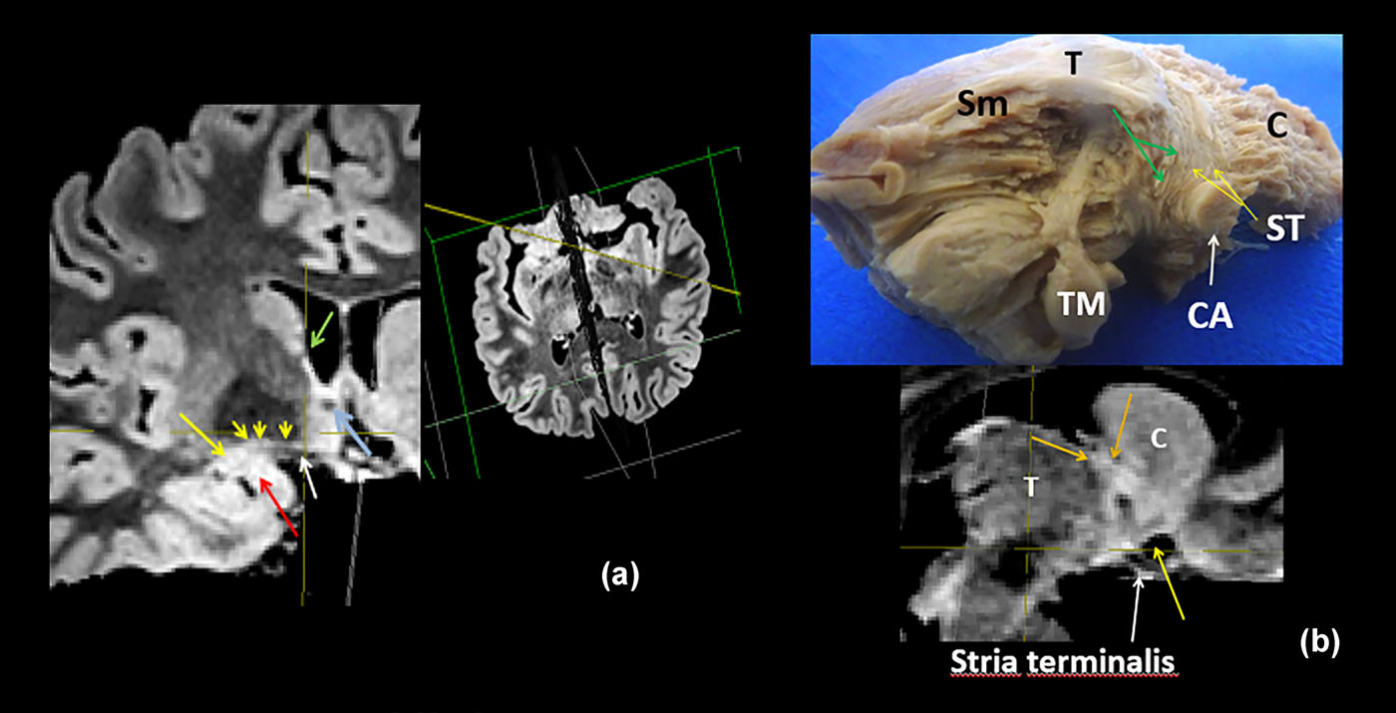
(a) MRI oblique plane of cut in our model to expose the continuity of the sublenticular extended amygdala (SLEA), from the centro‐medial amygdala to the bed nucleus of the stria terminalis (BNST). The obliquity of the cut is shown at the side—bigger yellow arrow, central nucleus of the amygdala; red arrow, medial nucleus of the amygdala; little yellow arrows, sublenticular extended amygdala with characteristic irregularity/discontinuity in human (Heimer et al., 2008); white arrow, optic chiasm and optic tract. The cut is almost parallel to them, but tilted posteriorly; light blue arrow, anterior commissure encircled by the BNST; green arrow, thalamostriate vein; extending downward from it, the dorsal bed nucleus (BNSTd). (b) Above—Fibers of the stria terminalis (ST) arrive at the region of the bed nucleus following the thalamo‐striate sulcus and divide in its pre‐ and postcommisural components (yellow arrows). They are accompanied by the supracapsular (dorsal) portion of the bed nucleus (BNSTd). T, thalamus; Sm, stria medullaris; TM, mammillary body; C, caudate nucleus; CA, anterior commissure; ST, stria terminalis. The stria terminalis and stria medullaris interlace their fibers around the commissure (green arrows) (Crosby et al., 1962; Jhonston, 1913). Below—MRI sagittal cut. The bed nucleus distinguishes for its high signal and seems accompanied by fibers of the pre‐ and post‐commissural components of the stria terminalis. white arrow, optic chiasm; yellow arrow, medial anterior perforated substance (APS); Orange arrows, stria terminalis pars dorsalis.
